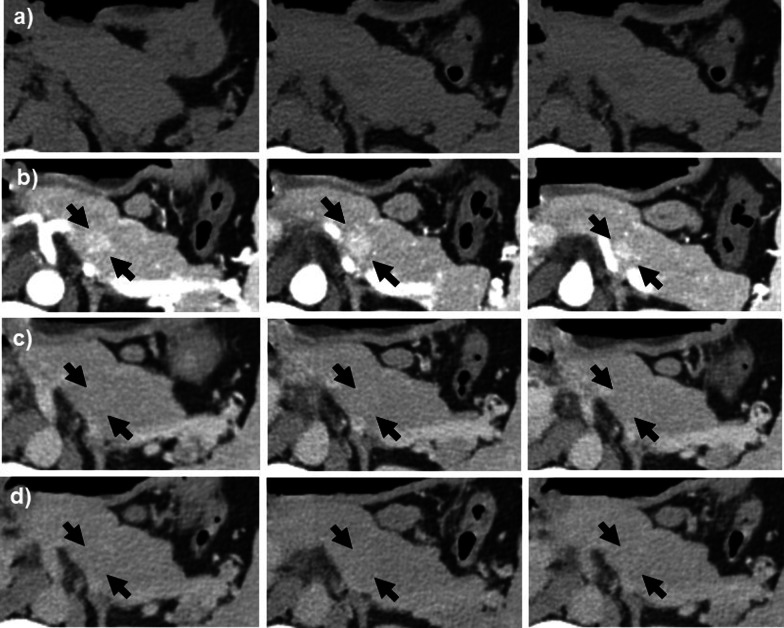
Contrast-enhanced CT of the abdomen. a Plain CT, b arterial phase, c portal phase, and d equilibrium phase. A 13-mm-long well-defined mass was found in the tail of the pancreas. The tumor showed slight low density compared with the pancreatic parenchyma on plain CT (a) and enhancement in the arterial phase and equal density with the pancreatic parenchyma in the portal and equilibrium phases on dynamic enhanced CT (black arrows in b–d). Tumor abutment to the splenic artery and vein without encasement was observed. No significantly enlarged regional lymph nodes and distant metastases were noted
